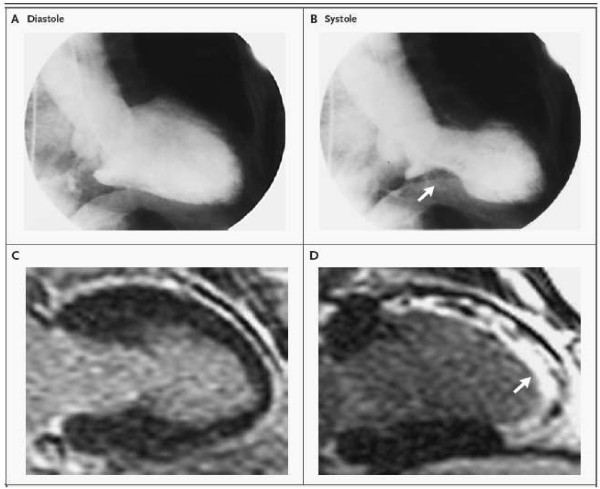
Left ventricle angiography during diastole (A) and systole (B) showing apical and mid ventricular wall motion abnormalities and hyperkinesis of the basal segment (arrow). MRI in long axis showing that the akinetic regions are hypoenhanced and dark suggesting the presence of viable myocardium (C). Reference after an acute myocardial infarction showing hyperenhancement indicative of necrosis. From reference (3) with permission.
